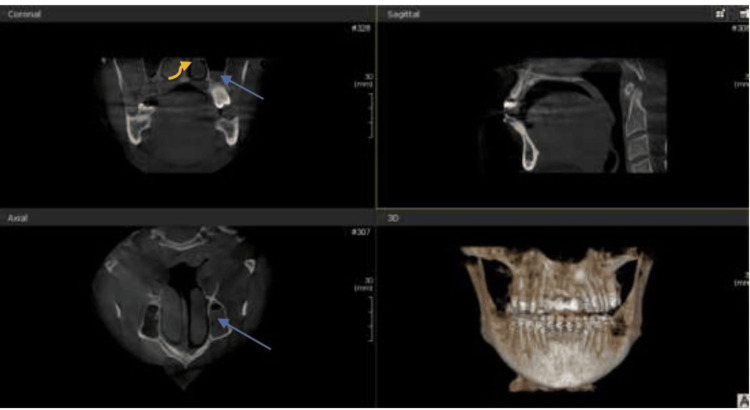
Left maxillary sinus opacification (blue arrows in both coronal and axial section) and deviated nasal septum (yellow curved arrow in coronal section) in a patient who presented for nerve treatment Coronal, axial, and sagittal CBCT images showing incidental maxillary and nasal findings in an orthodontic patient. CBCT: Cone-beam computed tomography
